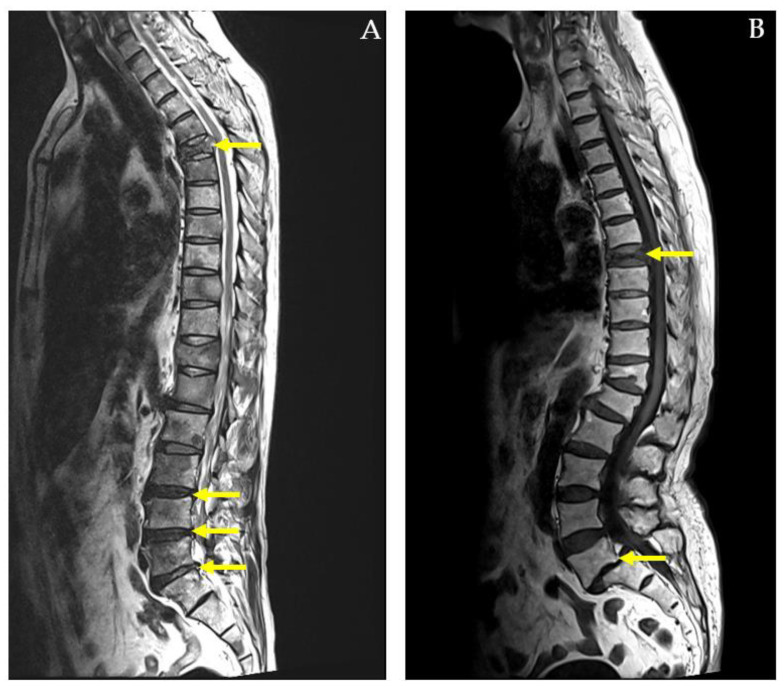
MRI whole-spine imaging demonstrating pathological compression fractures, herniated intervertebral discs (HIVDs), and spondylolisthesis indicated by yellow arrows. In (A) a 59-year-old male diagnosed with lung cancer and diffuse bony metastases affecting the cervical, thoracic, lumbar spine, sacrum, bilateral iliac bones, and right femoral head. The MRI reveals a pathological compression fracture of the T5 vertebral body with associated spinal cord compression. Additionally, herniated intervertebral discs (HIVDs) are present at the L3-L4, L4-L5, and L5-S1 levels. In (B) an 87-year-old female with a compression fracture at the T8 vertebral body. The MRI also shows L5-S1 spondylolytic spondylolisthesis with narrowing of bilateral neural foramina.
